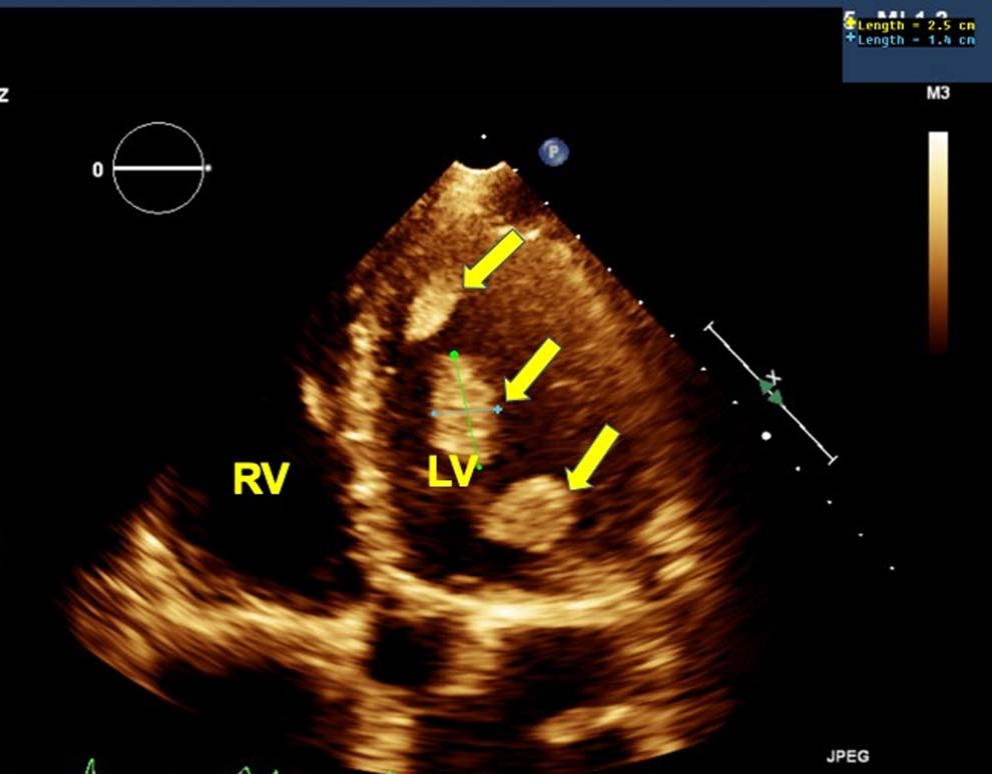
Transthoracic echocardiogram apical 4-chamber view demonstrates multiple left ventricle masses (arrows) with the largest mass measuring 2.5 cm × 1.5 cm. LV, left ventricle; RV, right ventricle.
